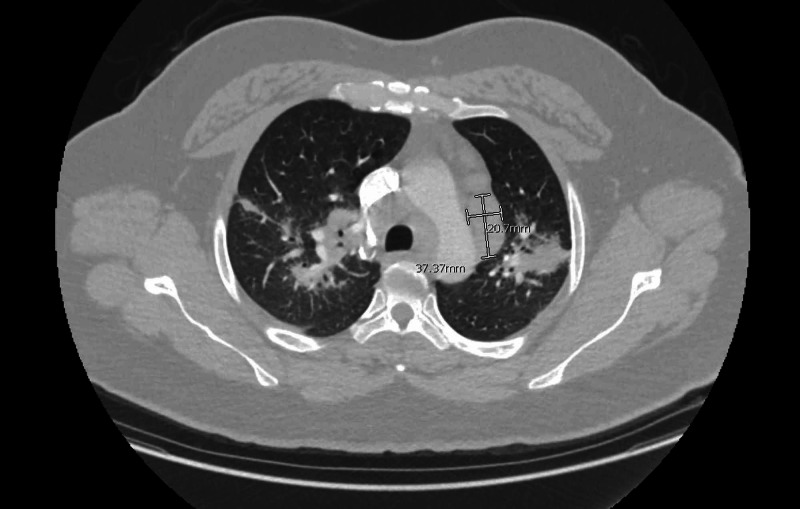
CT angiography chest transverse view showing enlarged left anteroposterior lymph node measuring 2.1 cm × 3.7 cm (dimensions marked in white).
